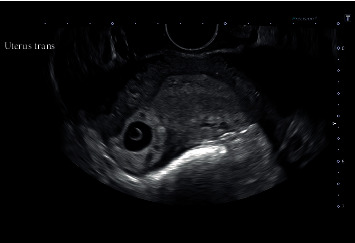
Ultrasound image of a right-sided cornual ectopic pregnancy.
